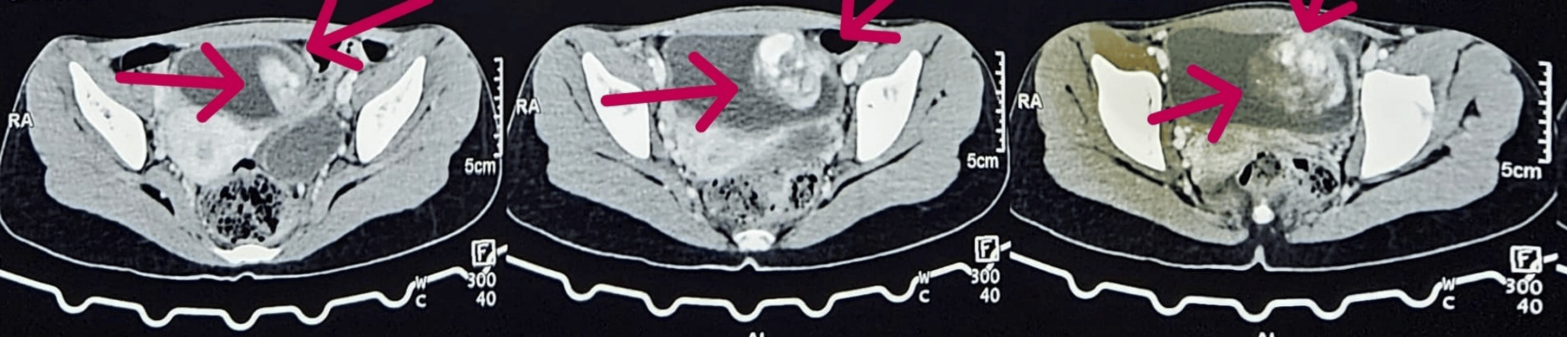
Contrast CT showing mass lesion arising from the left dome of the bladder.
